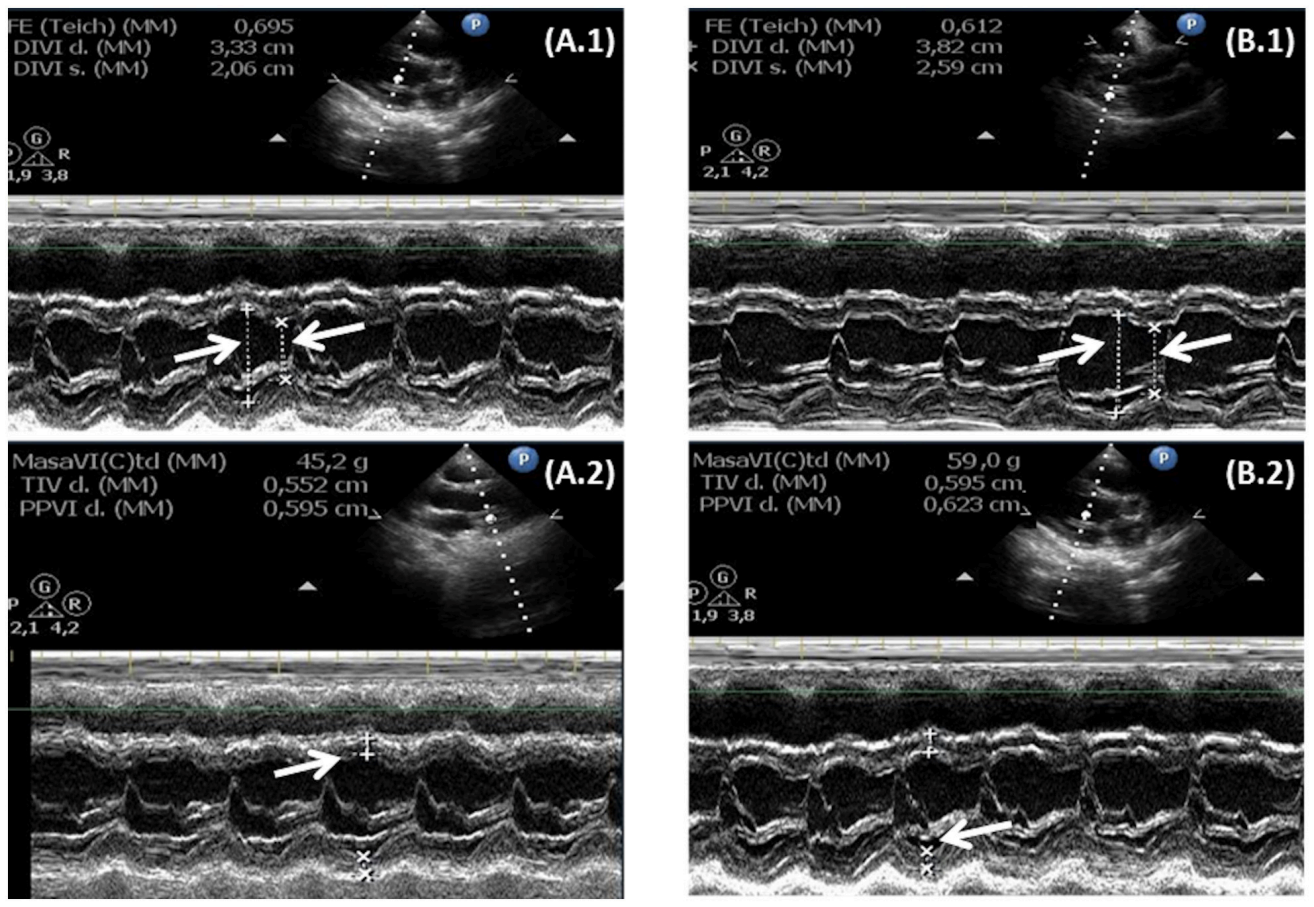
Image and data of left ventricular function and mass index (LVMI) measured by echocardiography mode M in GHD infants at baseline (A.1,A.2) and after 6 months with GH replacement therapy (B.1,B.2). Arrows indicate the systolic and diastolic diameter.
